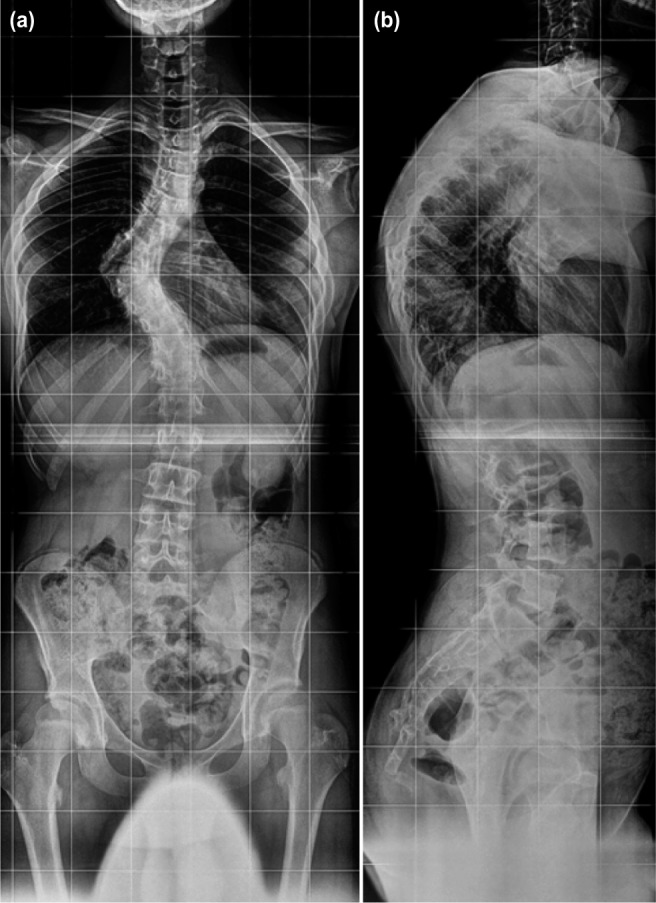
Standing whole spine radiographs showing Cobb angles of (a) sharp angular right-sided 64 degrees upper thoracic scoliosis (T5-T9), and (b) 74 degrees kyphosis (T4-T11).
